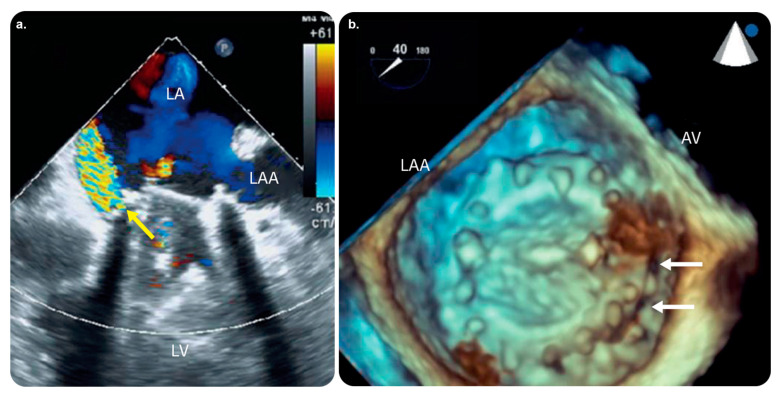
EchoNavigator software and its role in intuitive display. Preprocedural TEE. (a) 2D TEE color Doppler image showing severe paravalvular leakage (yellow arrow). (b) 3D TEE image showing dark holes between the prosthetic mitral valve and mitral annulus (white arrows) localized at the medial side of the anterior mitral annulus near the aortic valve. Abbreviations: AV, aortic valve; 3D, three-dimensional; 2D, two-dimensional; LA, left atrium; LAA, left atrial appendage; LV, left ventricle; TEE, transesophageal echocardiography. Reprinted from “Three-dimensional EchoNavigator system guided transcatheter closure of paravalvular leakage” by Kim et al. [70] with permission from the corresponding author and the publisher (Journal of Cardiovascular Imaging; Copyright 2019 [open access license]).
